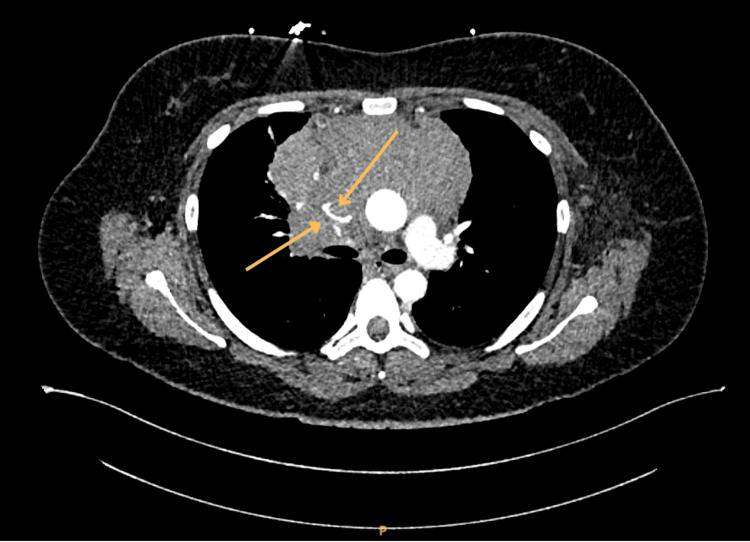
Axial CT aortogram showing a bulky anterior mediastinal mass with marked compression of the SVC (arrows), consistent with SVC obstruction CT: computed tomography, SVC: superior vena cava
